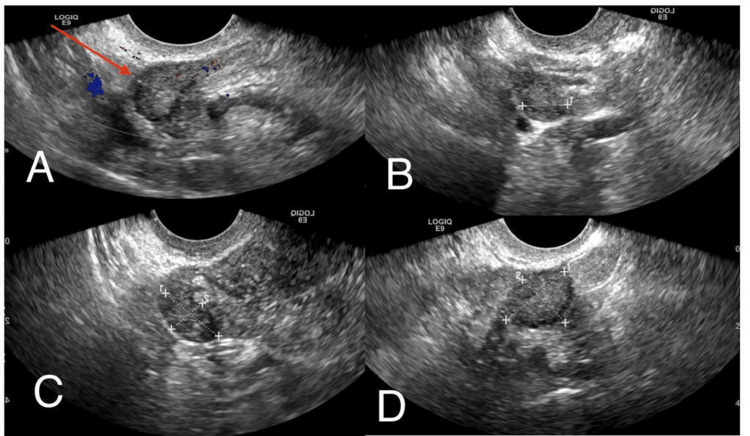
Transvaginal ultrasound showing a retroverted uterus with normal endometrial stripe thickness and a trace amount of free fluid in the endometrial cavity. Unremarkable ovaries. No free pelvic fluid. There is no evidence of ovarian, uterine, or adnexal mass at the moment of diagnosis.
